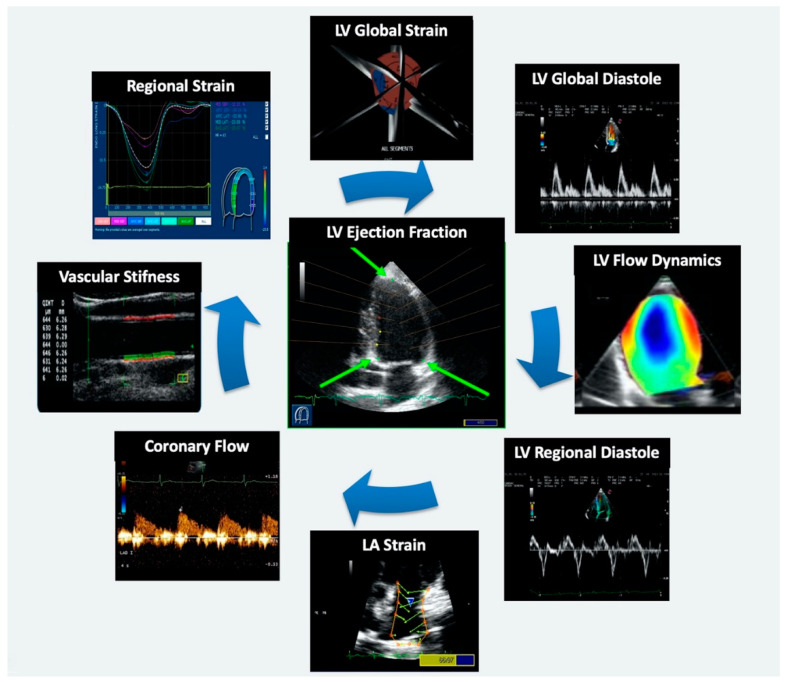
From standard echocardiography to new technologies. The incremental contribution of new echo technologies to provide valuable insights in the pathogenesis of cardiovascular disease, with distinct patterns of myocardial, vascular, and microcirculatory dysfunction. LV: left ventricle. LA: left atrial.
